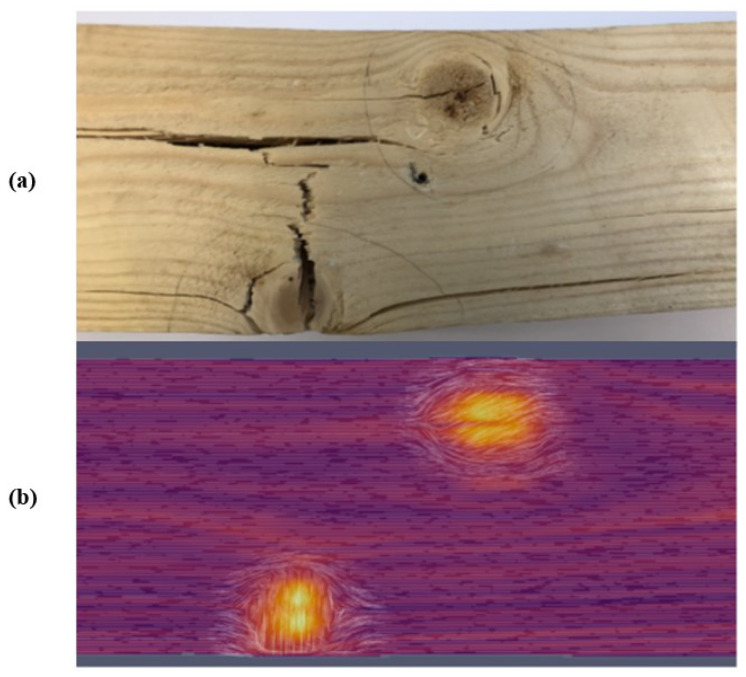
(a) The board was under test, with initial failure near the bottom-left knot, and (b) X-ray CT reconstruction at a depth of 10 mm. Source: [56].
